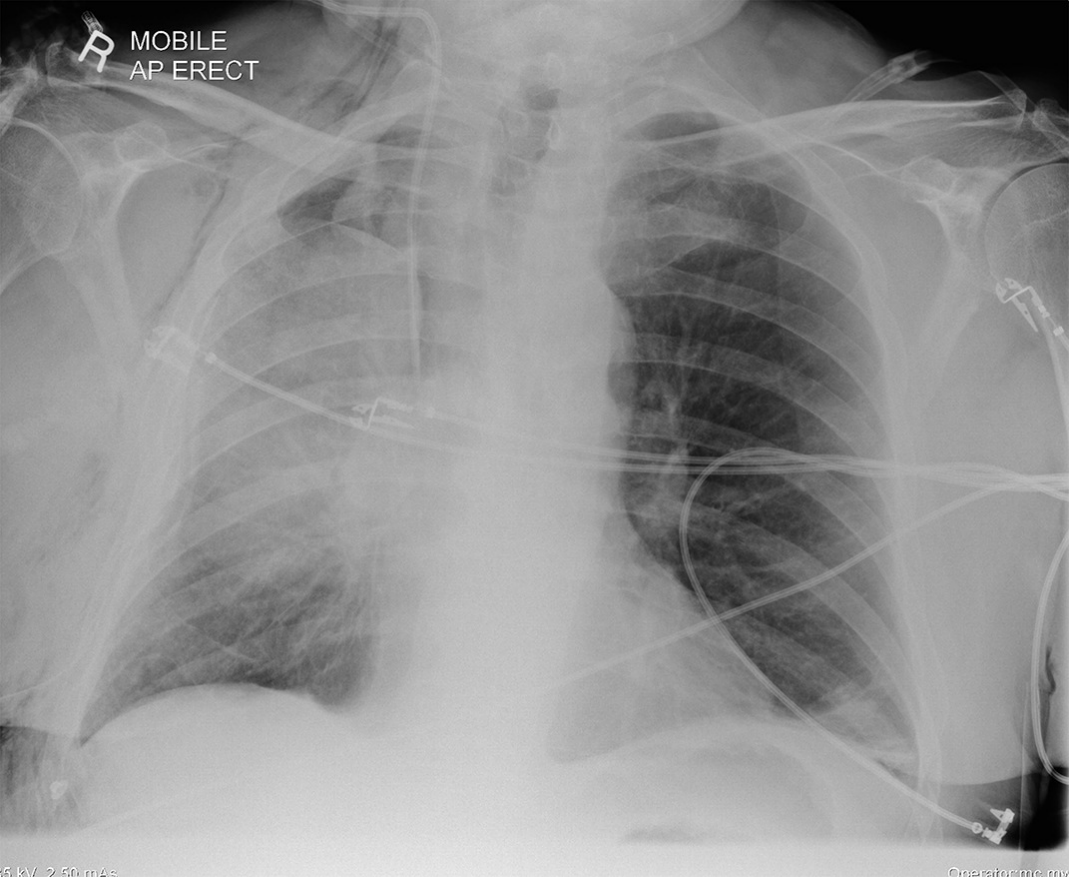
Chest radiograph performed 48 h postoperatively. There has been an increase in the right perihilar opacification, with well-defined superior and inferior margins formed by the boundaries of the torted right middle lobe; the diagnosis could have been suspected at this point. Surgical emphysema is seen throughout the soft tissues of the right chest wall. AP, anteroposterior.
